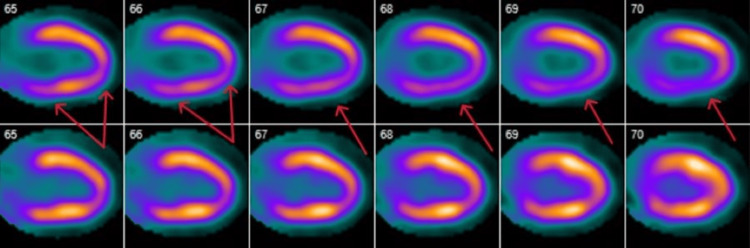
Vertical long-axis view of PET CT with arrows pointing to areas of ischemia in the apical region consistent with prior total left anterior descending artery occlusion.
